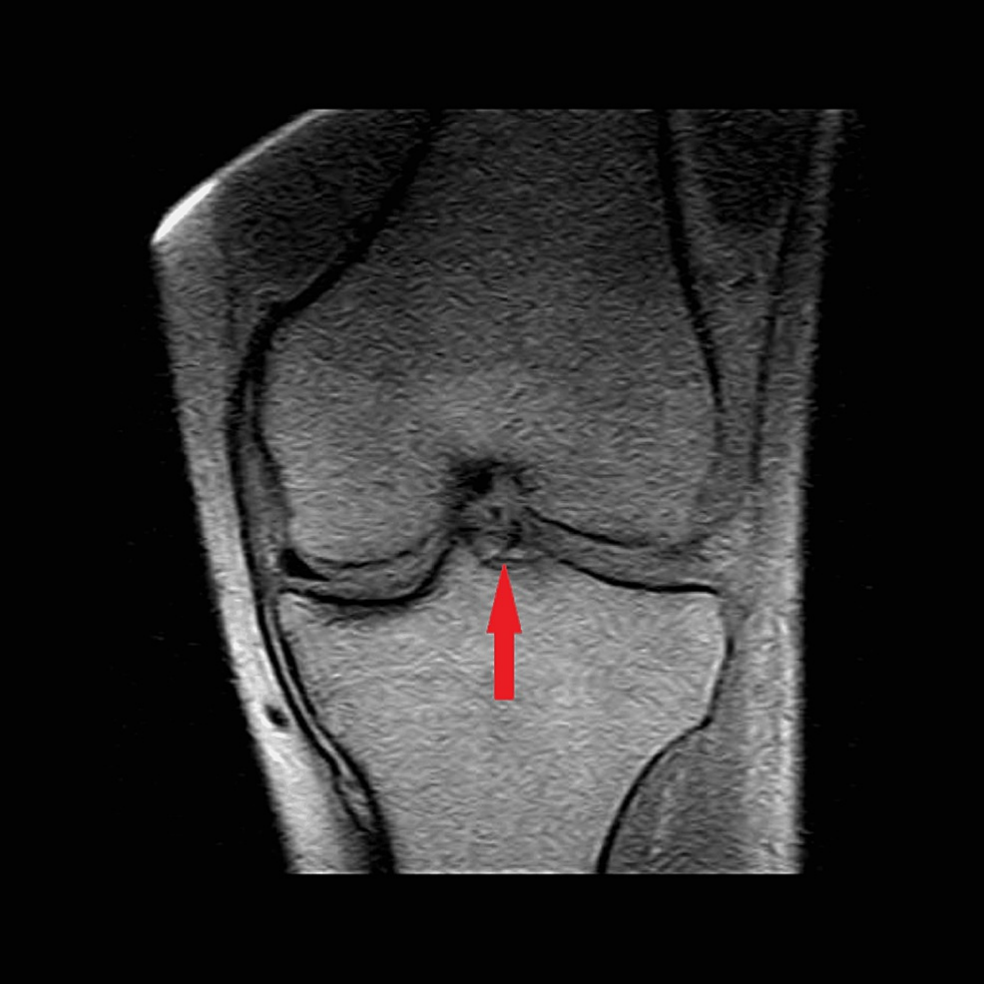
T1 coronal MRI left knee. Red arrow points toward the evidence of ligament damage. MRI: magnetic resonance imaging
